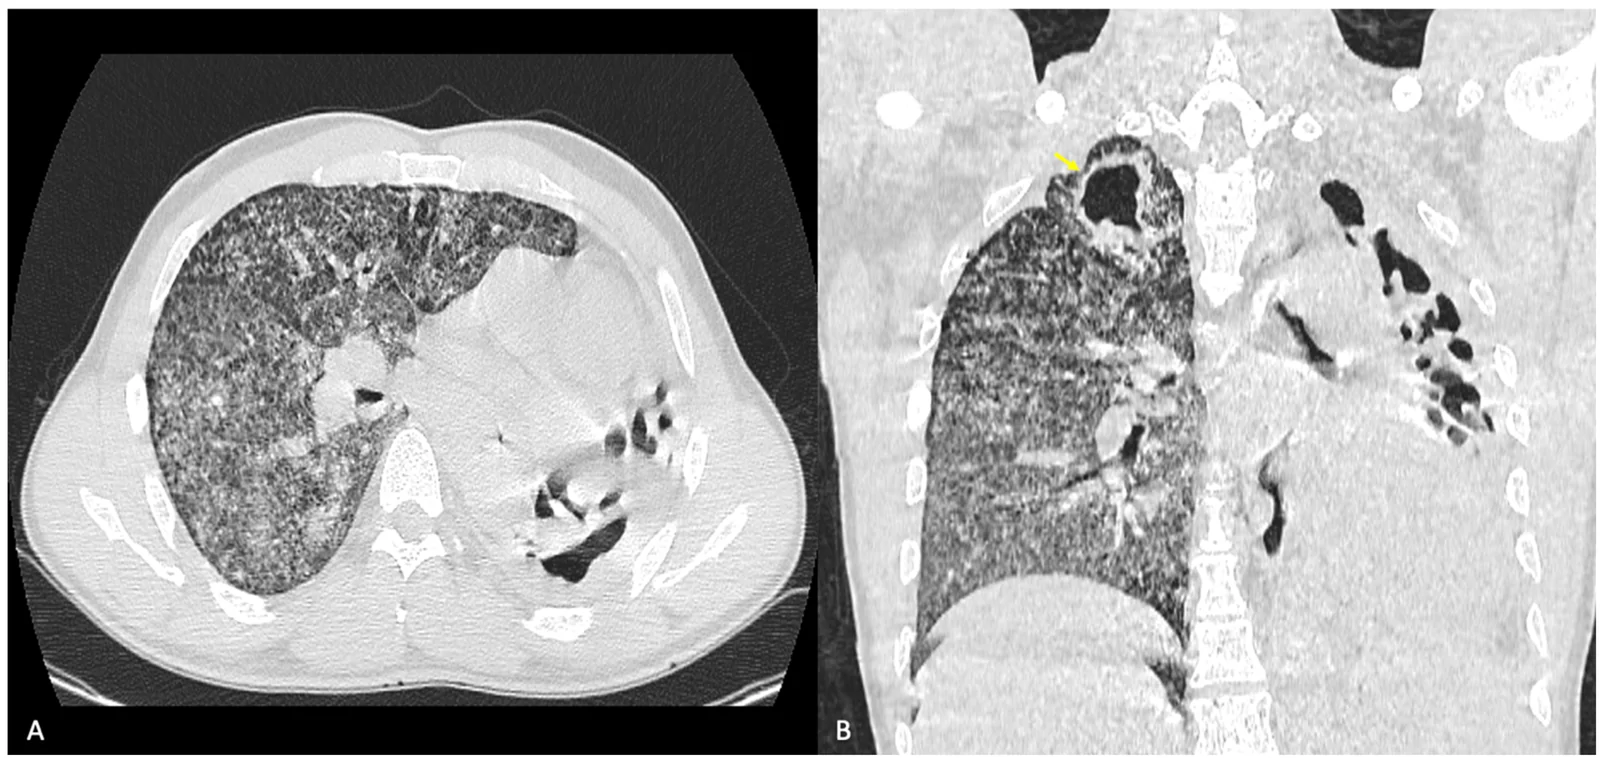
HRCT, axial (A) and coronal plane (B). A case of miliary TB presenting as countless 1–3 mm nodules randomly distributed in the entire right lung. In this case, other typical TB lung parenchymal abnormalities are present, as the extended cavitated pulmonary consolidations in the left lung and a tuberculoma in the upper right lobe ((B), yellow arrow).
